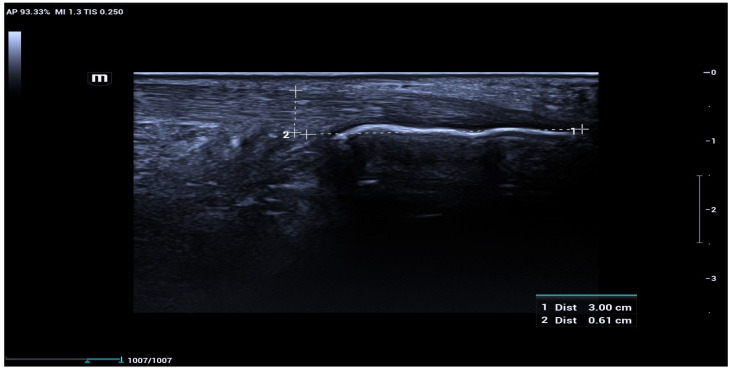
Measurement of Achilles tendon thickness. Longitudinal grayscale ultrasonographic image demonstrating measurement of Achilles tendon thickness performed 3 cm proximal to the calcaneal insertion. The measurement was obtained with the patient in the prone position and the ankle in a neutral position, ensuring minimal transducer pressure.
